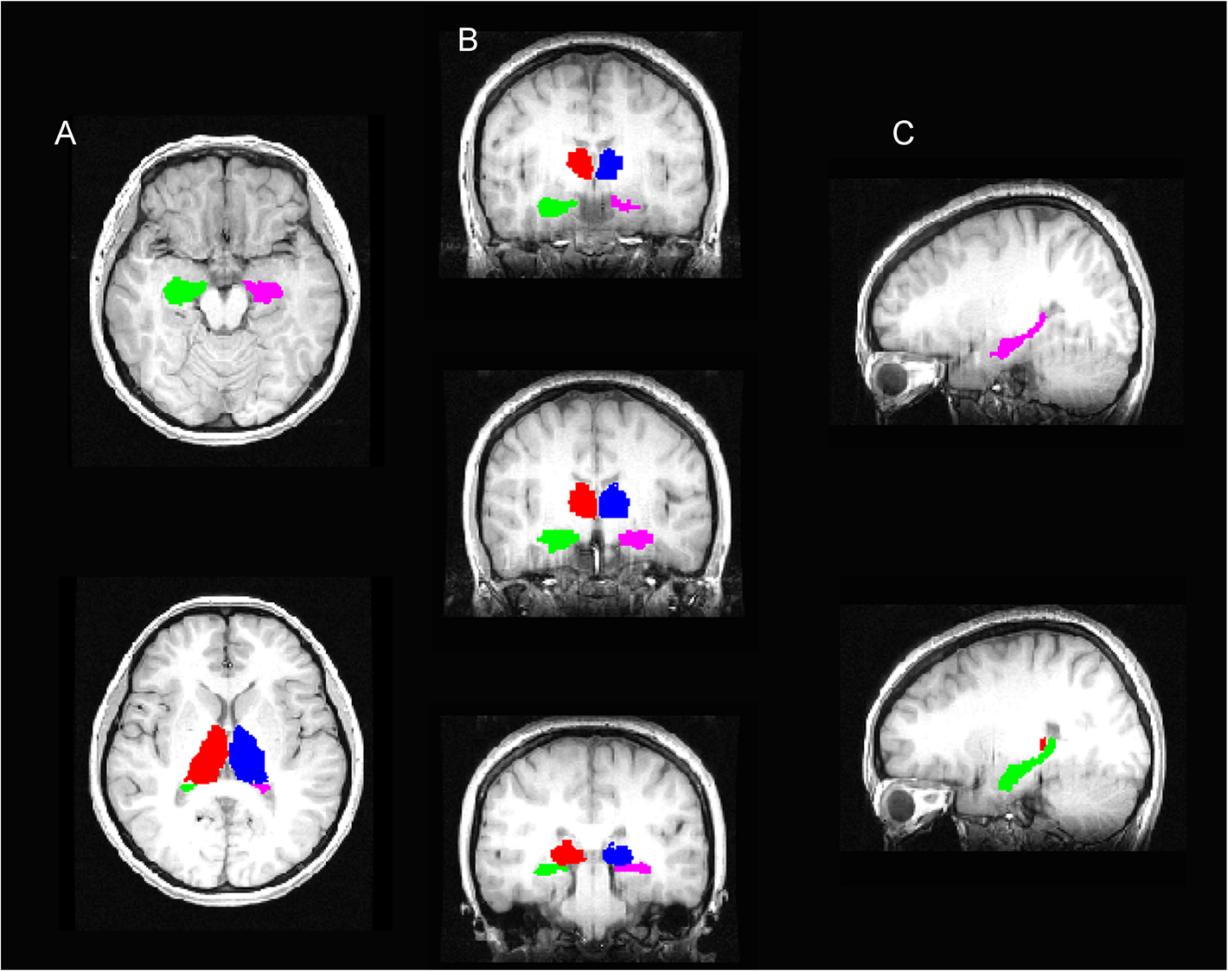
The segmented hippocampus and thalamus are shown on the patient’s MRI. A: The segmented hippocampus and thalamus are in a transverse section. B: From the anterior to posterior view, the segmented hippocampus and thalamus are in a coronal section. C: The bilateral hippocampi are segmented in a sagittal section (HS lateral, up; Contralateral, down)
